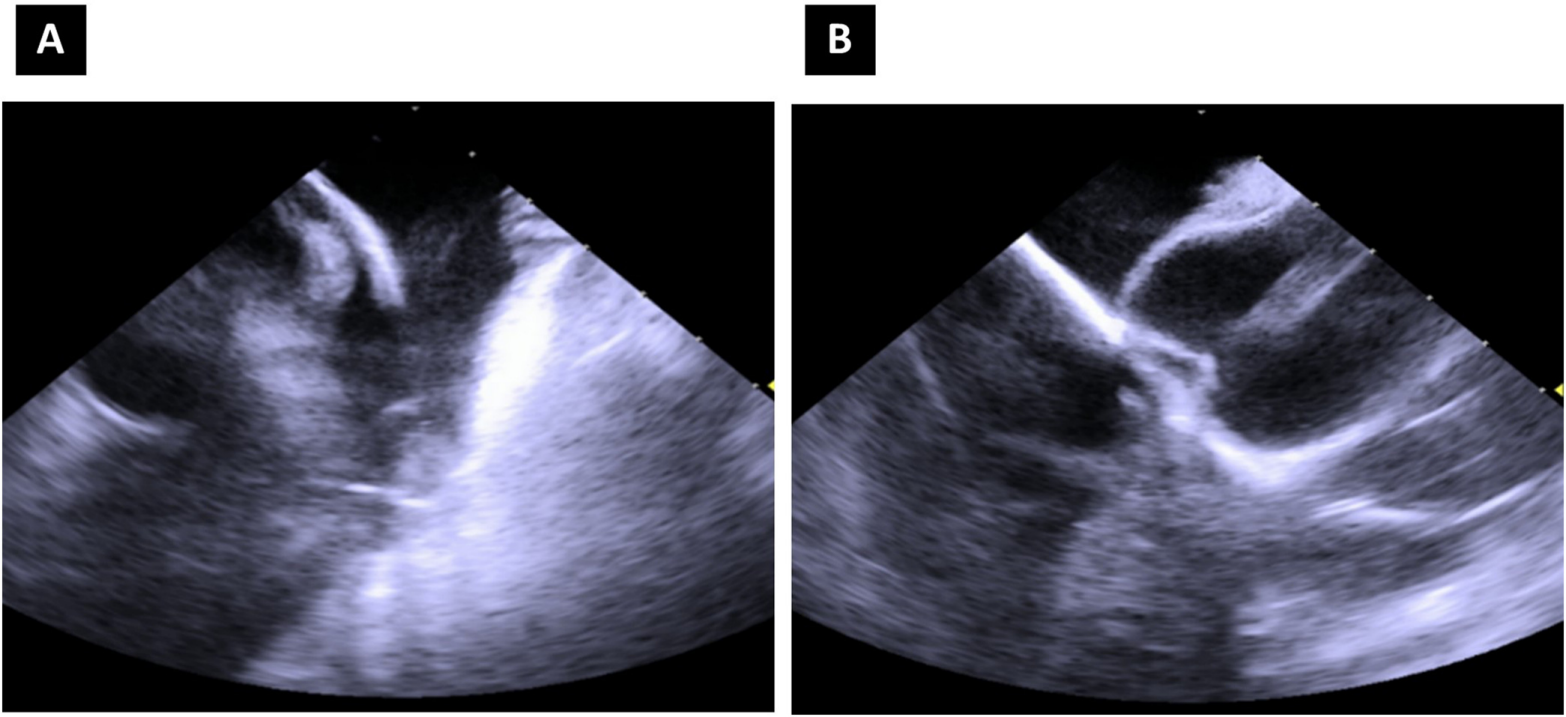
Intracardiac echocardiography image of the pentaspline catheter in “basket” configuration, right ventricular outflow tract view (A) and mid-right atrium—“home” view (B).
